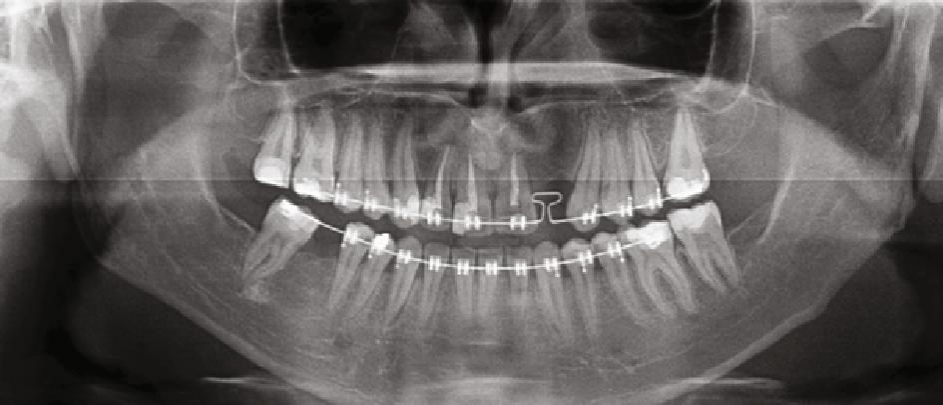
Panoramic radiograph of the patient before the orthognathic surgery.
